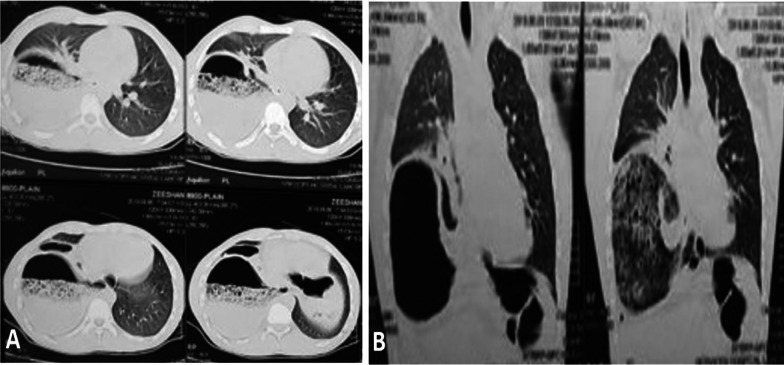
Contrast enhanced CT images of chest and abdomen visualized the loss of right lung volume along with stomach and bowel loops herniating into the right hemithorax. A Axial view of CT scan of chest and abdomen showed atelectatic changes in lung, normal heart size and hollow viscus on right with air and fluid alongside the enlarged liver. B Coronal view presented large cavitating lesion below right lung with loss of right lung volume and opacifying with contrast
